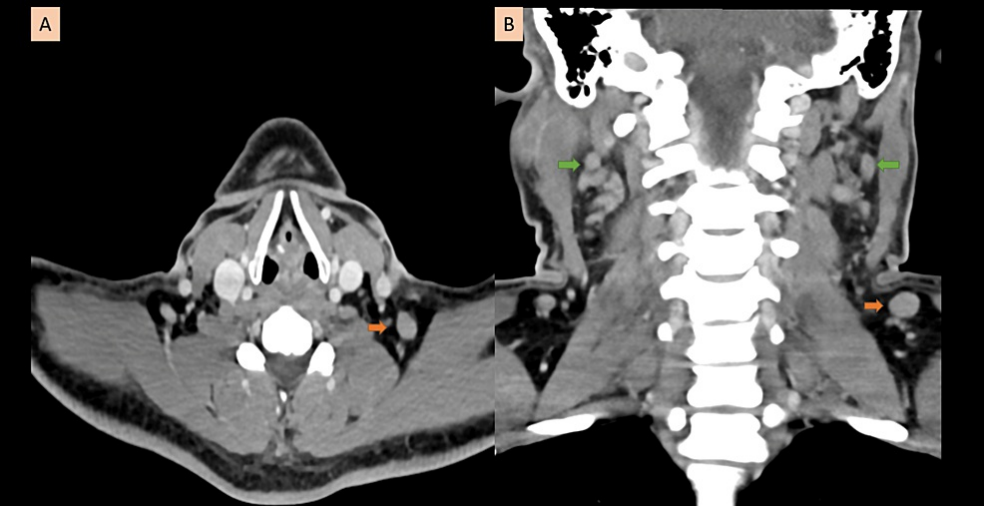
Axial (A) and coronal (B) computed tomography of the neck with contrast show left supraclavicular enlarged lymph nodes but no necrosis or calcification (orange arrow). Multiple bilateral reactive deep cervical lymph nodes can be seen (green arrow).
